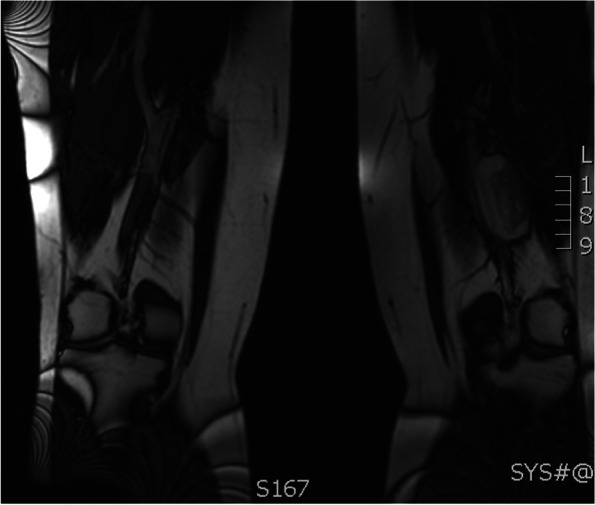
MRI (Coronal view) demonstrating saccular aneurysmal dilation measuring 43 × 31 × 68 mm
